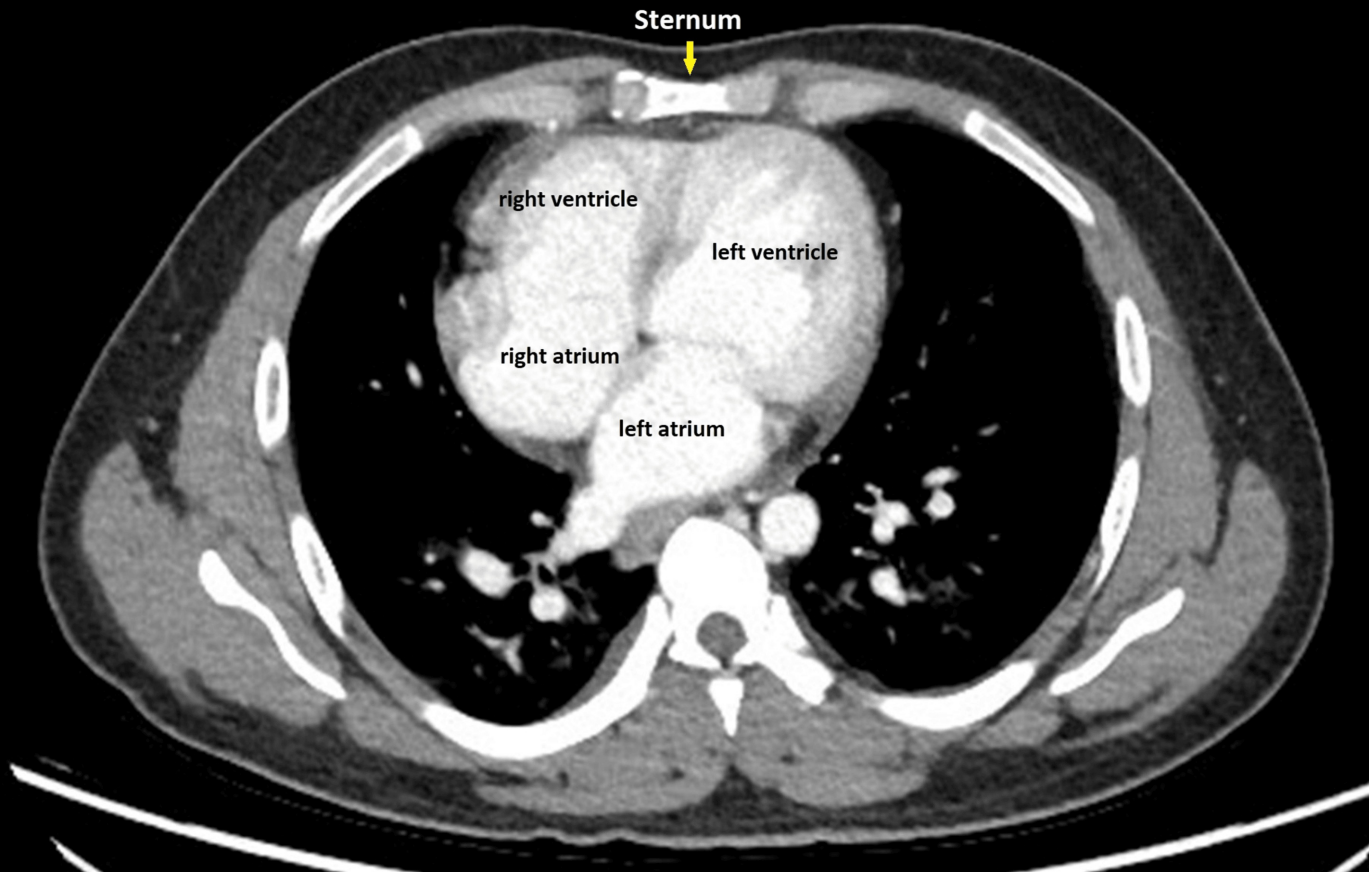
Axial CT scan of the chest showing an anticlockwise rotated heart CT: computed tomography
